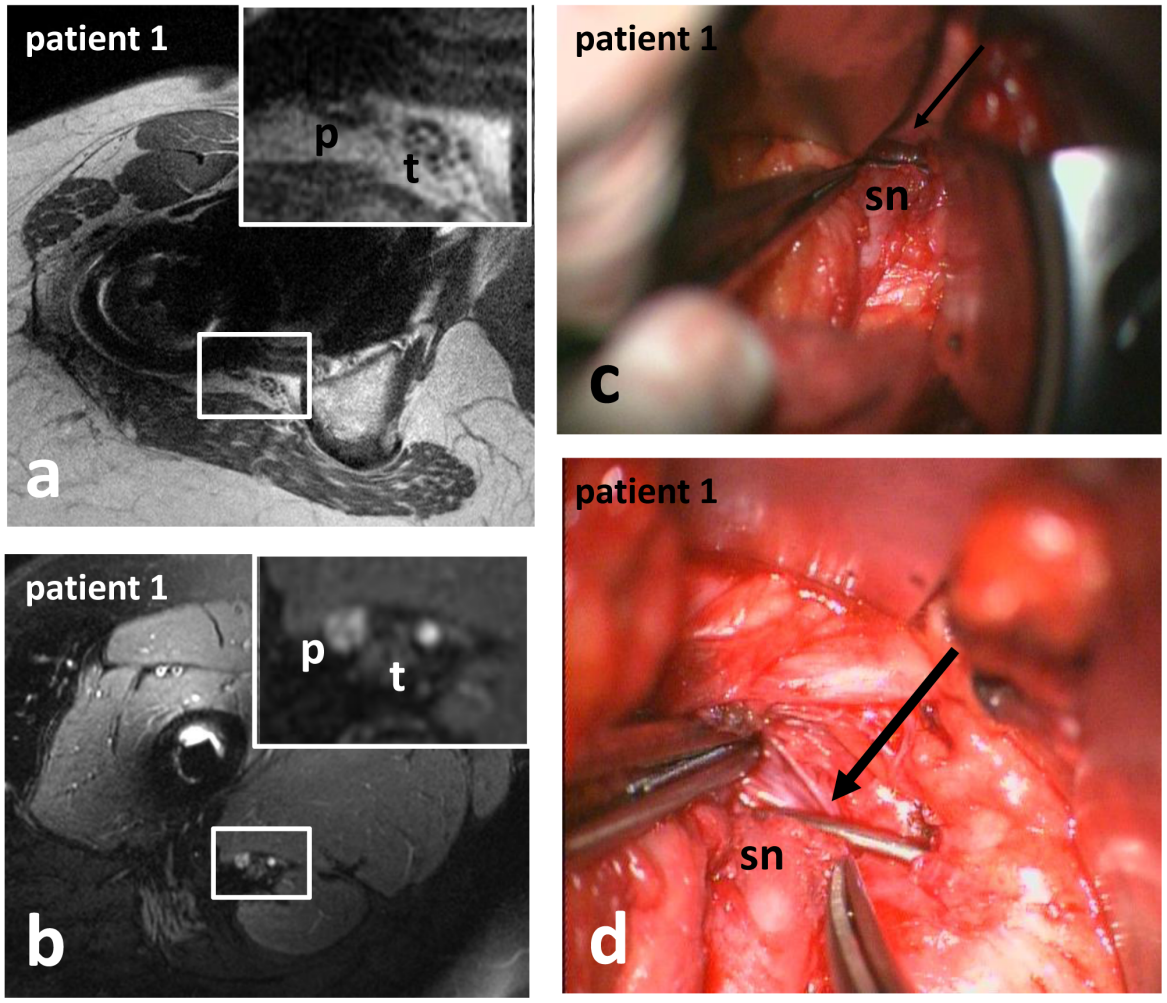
Constriction of the sciatic nerve by surgical material in patient 1 (a, b: siMRN; c, d: intraoperative photographs). T1-weighted sequences, being less vulnerable to susceptibility artifacts, revealed constriction of the peroneal portion of the subtrochanteric sciatic nerve by a cerclage (a). Below the constriction, the peroneal portion of the sciatic nerve exhibited a pathological T2-signal-increase (b). The constriction of the sciatic nerve by a cerclage (arrow in c, d) was validated intraoperatively. Abbreviations: p: peroneal portion of the sciatic nerve or peroneal nerve; sn: sciatic nerve; t: tibial portion of the sciatic nerve or tibial nerve.
